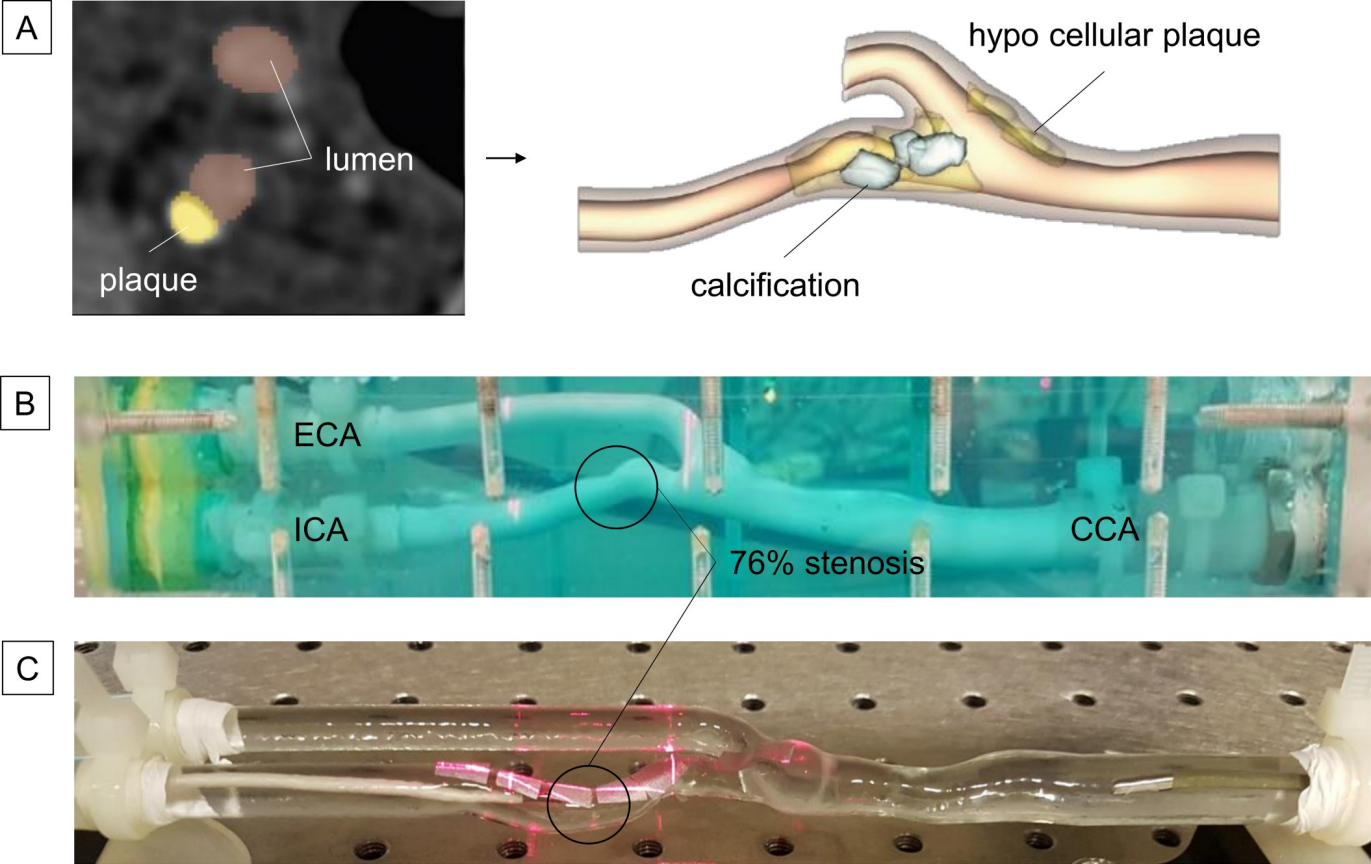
Patient-specific model. (A) CTA images of the carotid bifurcation from a 75 year old male were processed by Iannaccone et al.[20] in order to obtain a patient-specific model of the plaque-free stenosed vessel. The compliant (B) and stiff (C) models used for the hydraulic tests replicate the geometry of the patient-specific common carotid artery (CCA), external carotid artery (ECA), and internal carotid artery (ICA) where the 76% area stenosis (circled) is located.
